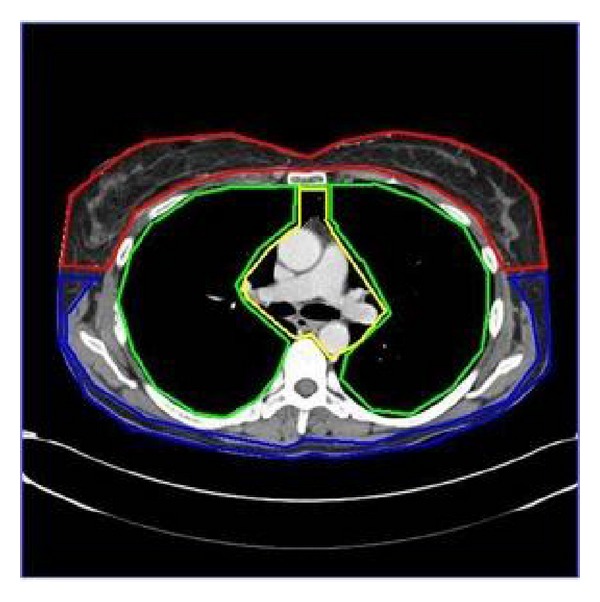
Axial contrast CT image of chest. Image delineates the six regions used in the qualitative comparison of the scans with and without the breast shield in patients. The six regions are anterior thoracic wall (red), lateral and posterior wall (blue), lung parenchyma [10], mediastinum, heart, and aorta and great vessels (all three within yellow).
